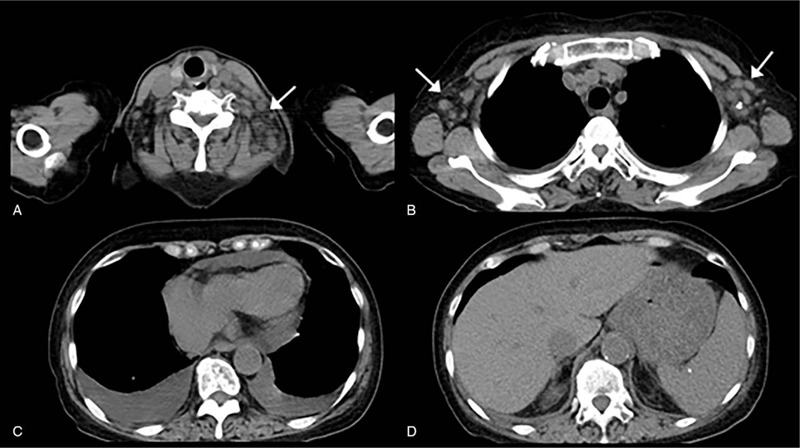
Computed tomography scan reveals cervical lymphadenopathy (A, arrow), bilateral axillary lymphadenopathy (B, arrow), bilateral pleural effusion, pericardial effusion (C), and hepato-splenomegaly (D).
